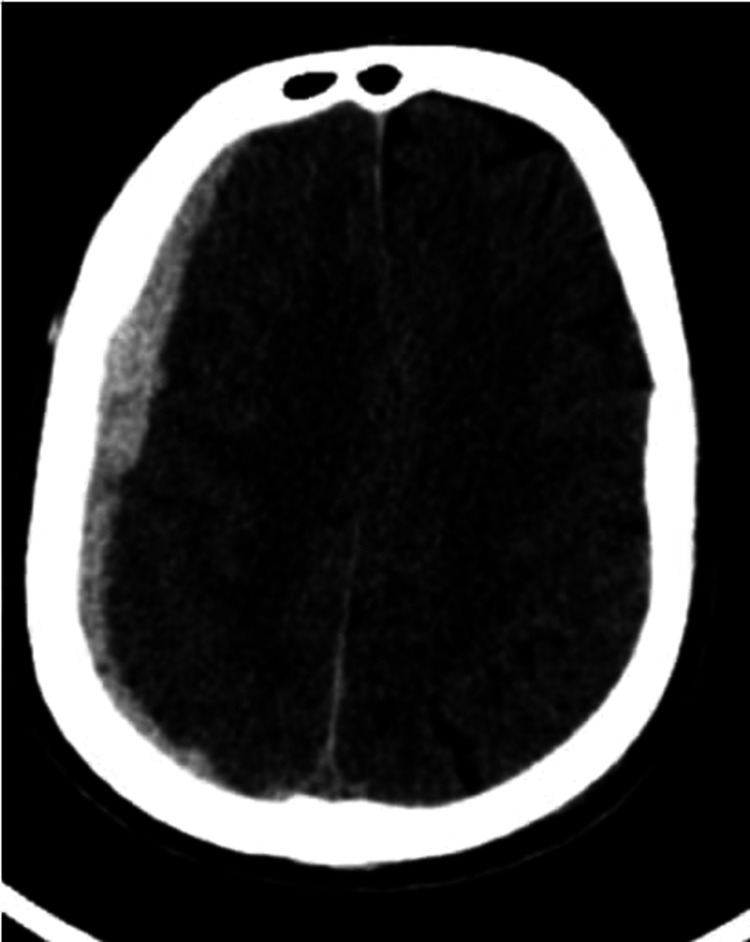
Axial NECT shows a subdural hemorrhage in the right cerebral hemisphere NECT: non-contrast-enhanced computed tomography
